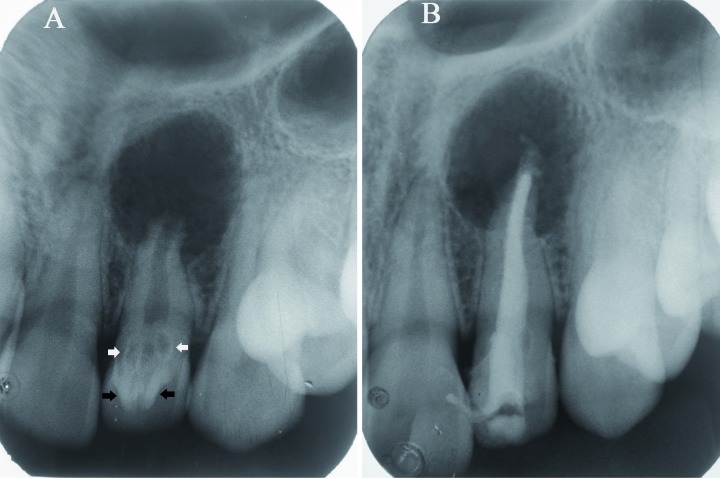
A) Intraoral periapical radiograph (IOPA) showing two dense invaginatus (white arrows) below two talon cusps (black arrows) and a well defined radiolucency in relation to 22; B) Post-obturation radiograph.
